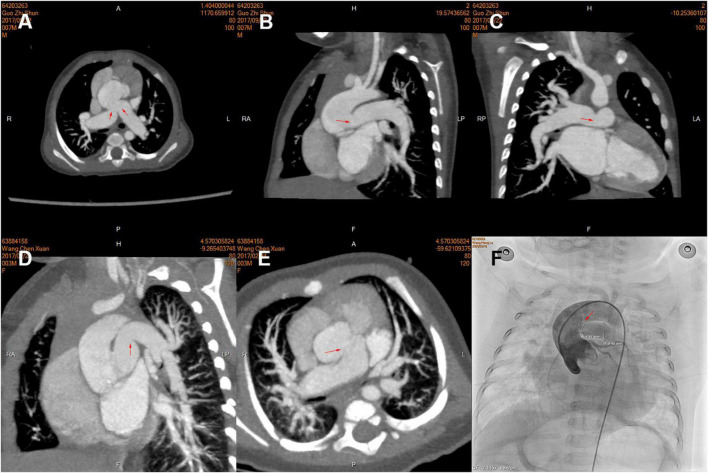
Pre-operative computed tomography (CT) angiography and cardiac catheterization showing branch pulmonary artery (PA) morphology of the different types of truncus arteriosus (TA), including the proximal and distal in three patients. (A–C) Type A2; (D,E) Type A1; (F) Type A3. The opening of branch PAs is labeled by red arrows.
